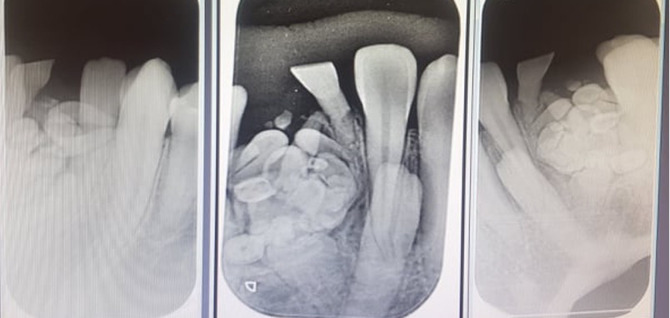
periapical radiography showing mixed radio-opaque and radiolucent lesion in the region of 71, 42 surrounded by a thin radiolucent band
